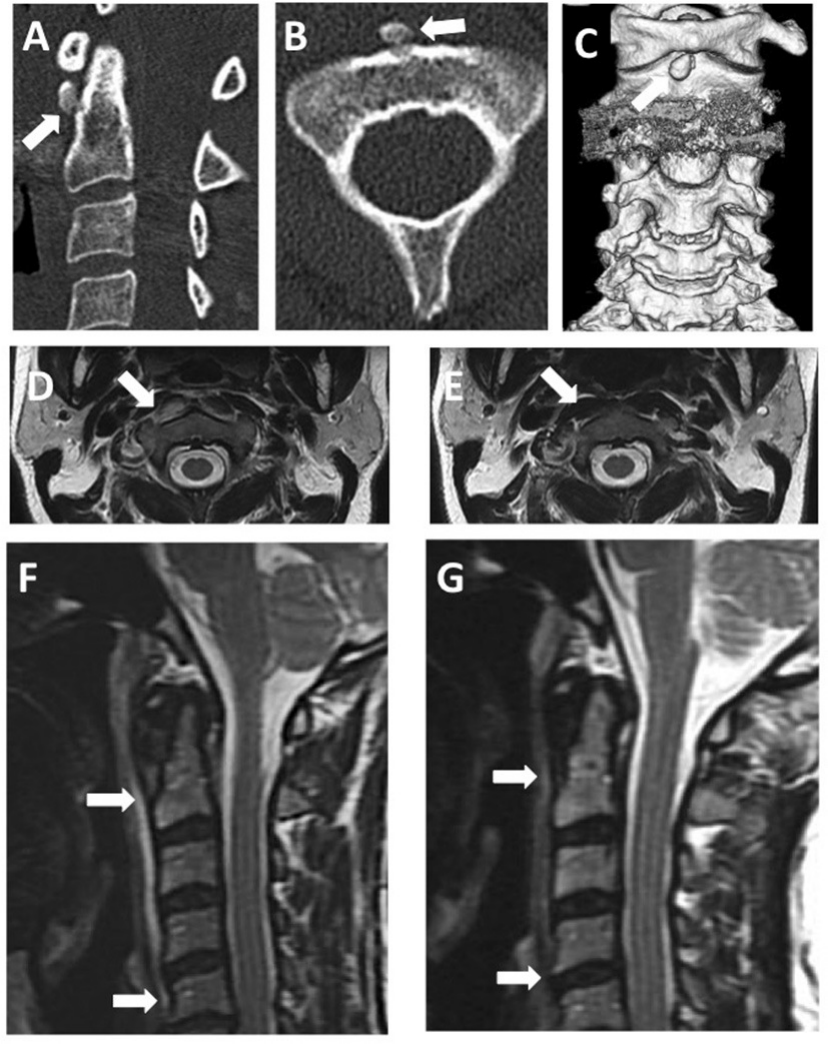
Images of acute calcific retropharyngeal tendinitis. (A) Sagittal cervical CT. (B) Axial cervical CT. (C) Three-dimensional reconstruction of cervical CT images. All cervical CT scans reveal nodular calcification in front of the C1–C2 vertebra (arrow). (D,F) T2-weighted cervical MRI before treatment. (E,G) T2-weighted cervical MRI after treatment. Before treatment, MRI reveals swelling and prevertebral edema (arrows). After treatment, swelling and edema have disappeared.
